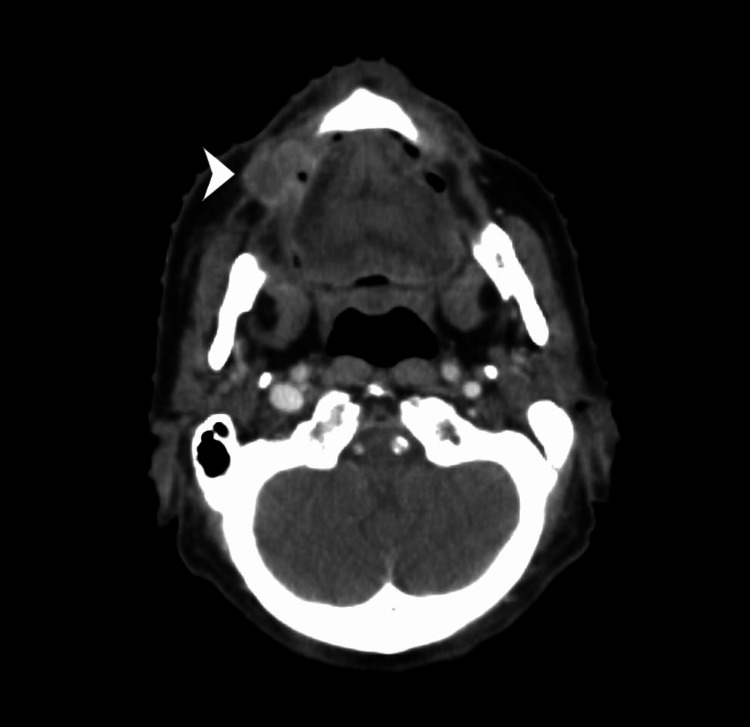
Whole body PET CT image with CT section of tumour (white arrow head is showing the tumour).
